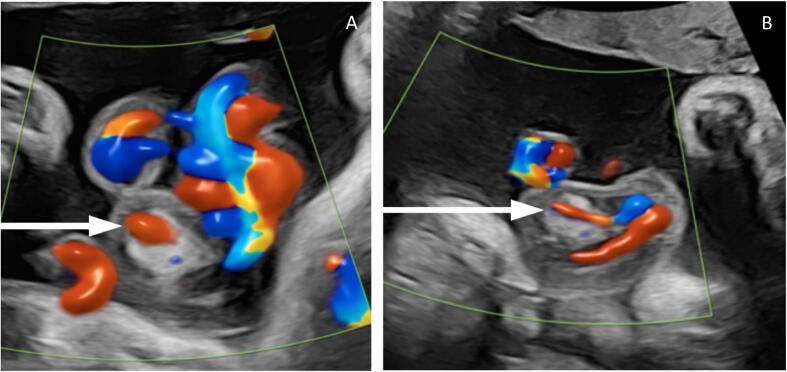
Colour Doppler ultrasound images demonstrating an umbilical cord hemangioma with a vessel seen within the lesion (indicated by arrows).
